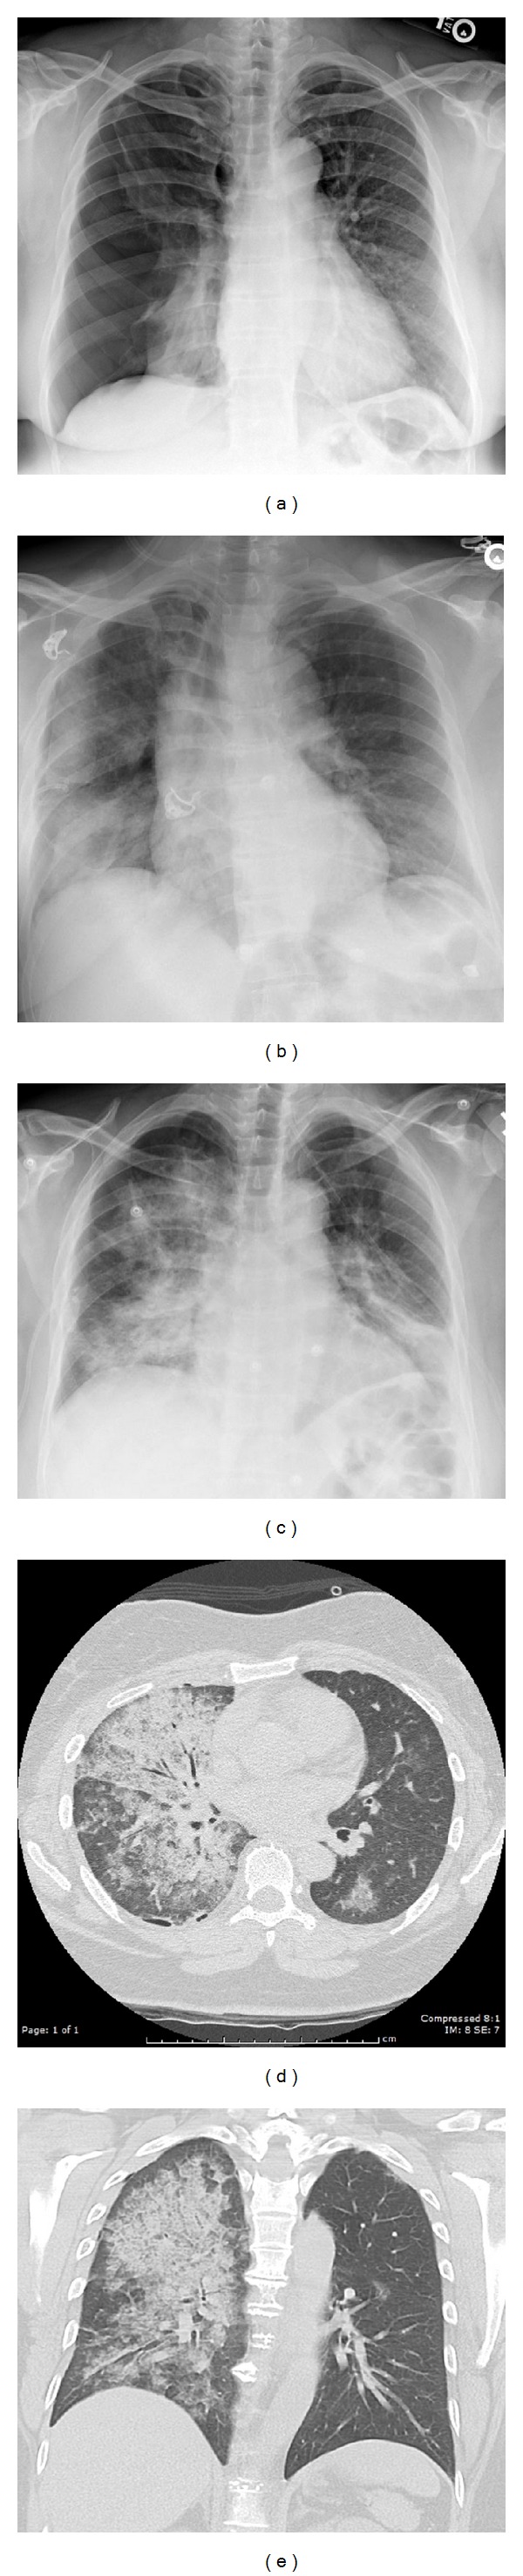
Selected case images. (a) Upright PA/lateral X-ray showing right sided large pneumothorax, (b) portable upright AP X-ray immediate postdecompression, (c) upright PA/lateral showing interval progression of REPE on day 2, (d) representative axial section (expiratory view, high resolution CT), and (e) representative coronal CT reconstruction.
